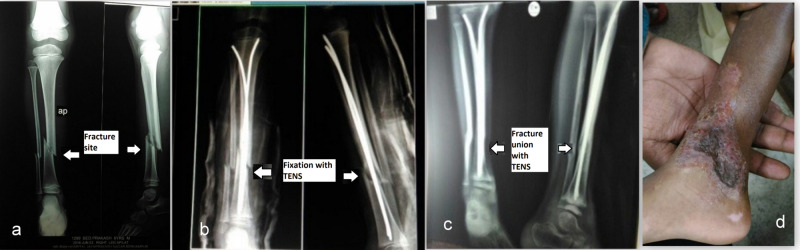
Tibia and fibula fracture managed with TEN a. Fracture of both bone legs preoperative radiograph, b. Immediate postoperative radiograph showing TEN, c. Seven months postoperative radiograph showing a united fracture, d. Clinical photo showing healed wound over the medial aspect ankle TEN: titanium elastic nail
